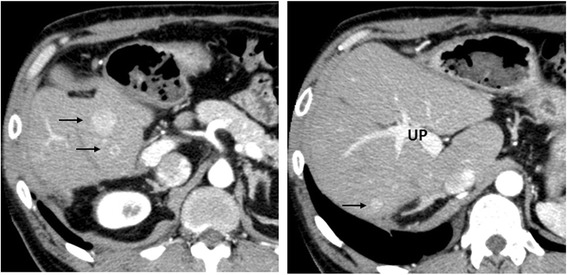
Computed tomography images of the initial recurrent lesions. Three well-enhanced masses in segment 4 are observed (*arrow*). UP umbilical portion of the left portal vein
